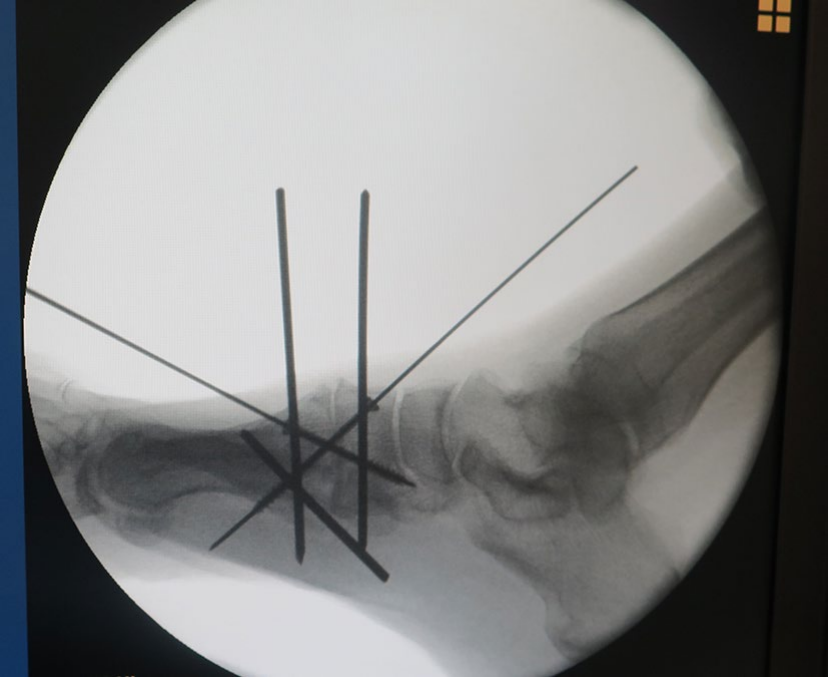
After the sonographic view of the correct position (Fig. 2a) of the sesamoid and FHL, one or two screws are placed through the tarsometatarsal (TMT) joint previous to the dorsomedial plate fixation
